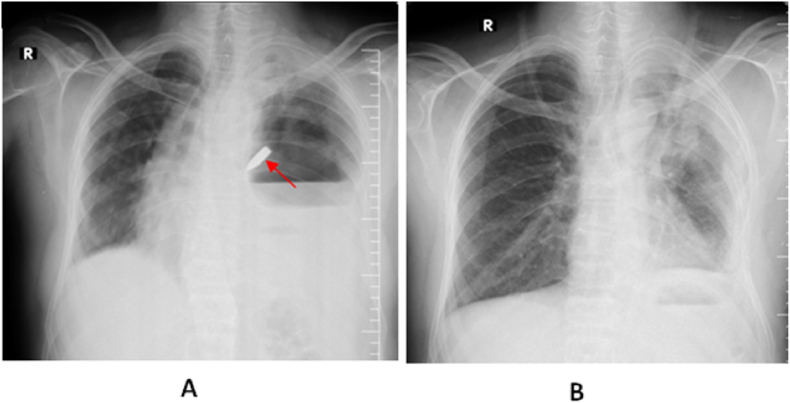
A- 3-mm radiopaque foreign body (red arrow) is seen in the left main bronchus on a chest radiograph. The left hemithorax also has an air-fluid level comparable to empyema.B- x-ray after spontaneous bullet expectoration. (For interpretation of the references to colour in this figure legend, the reader is referred to the Web version of this article.)
